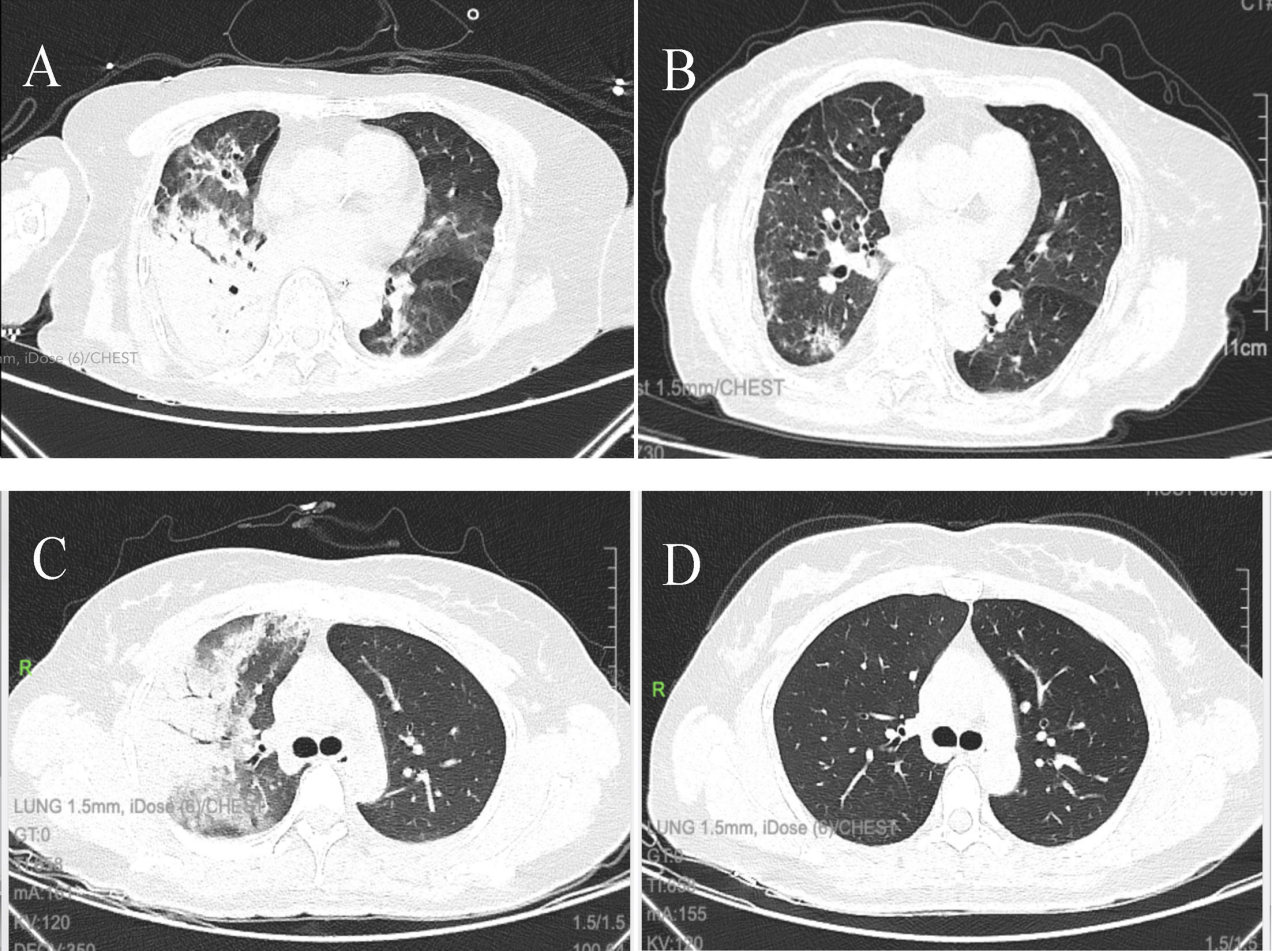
Chest computed tomography (CT) findings of *Chlamydia
